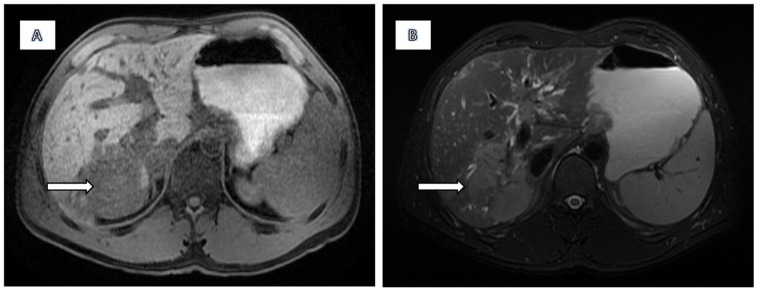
The lesion is moderately hypointense on T1-weighted images (A) and moderately hyperintense on T2 fat-suppressed sequences (B).
